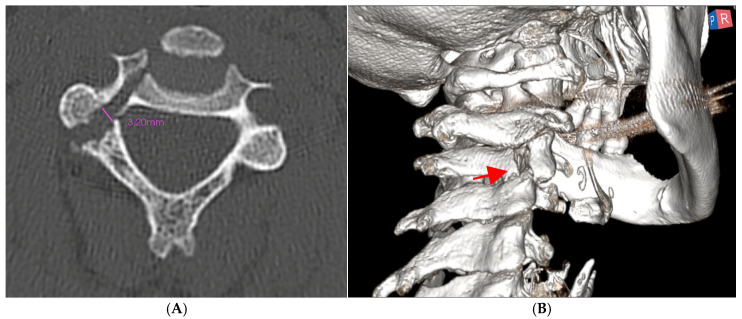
(A) axial and (B) 3D reconstruction of CT scans following 3 months of conservative treatment with a rigid collar. The progression of the distance between the heads of the fractures prompted the indication for surgical treatment. The red arrow indicates the fracture line.
